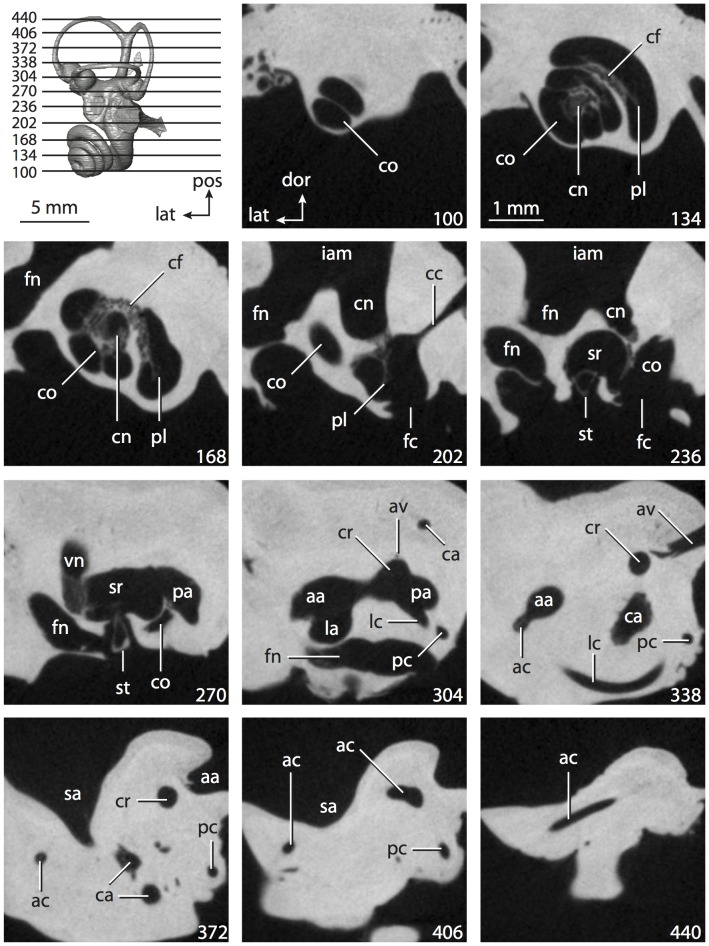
CT slices through ear region of*Sus scrofa*. Abbreviations listed at the end of the Materials and Methods section.
